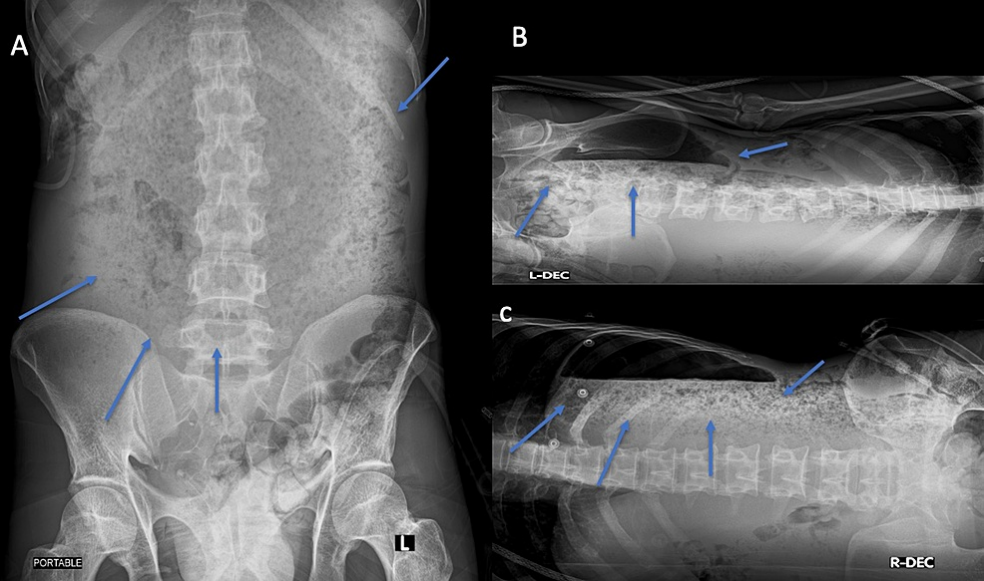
Supine (A) and lateral decubitus (B and C) abdominal x-rays show diffuse innumerable air foci (pointed by the blue arrows) mainly in the center of the abdomen (A) that corresponds to free air in the lateral decubitus (B and C) measuring around 15 x 5.8 cm (pointed by the blue arrows).
